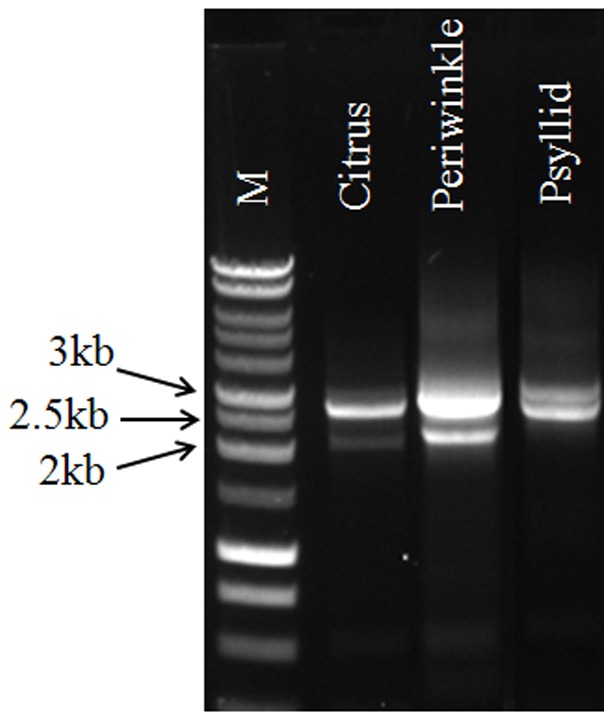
PCR products amplified from Las positive citrus, periwinkle and psyllid samples using primer LJ793/LJ795. M: 1 kb DNA ladder (Promega, Madison, USA); The CT value for three isolates by Taqman real-time PCR using 16 s rRNA gene based primers are: citrus, 19.95; periwinkle, 18.58; and psyllid, 18.26.
